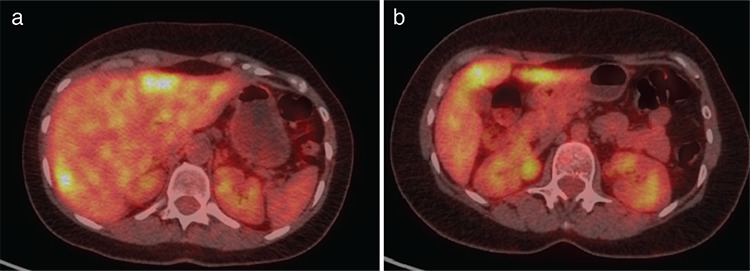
A restaging PET/CT of the same patient, showing progression 2 months after RFA with new liver lesion and increased size and activity of the ablated lesions. a) Fused axial post treatment image on the level of liver segments 2 and 7, showing metabolically active liver lesions respectively with SUV_max_ 5.5 and SUV_max_ 5.8. b) Fused axial post treatment image on the level of liver segment 4b, showing a new metastatic lesion with SUV_max_ 4.7 PET/CT: Positron emission tomography/computed tomography, RFA: Radiofrequency ablation, SUV_max_: Maximum standard uptake value
